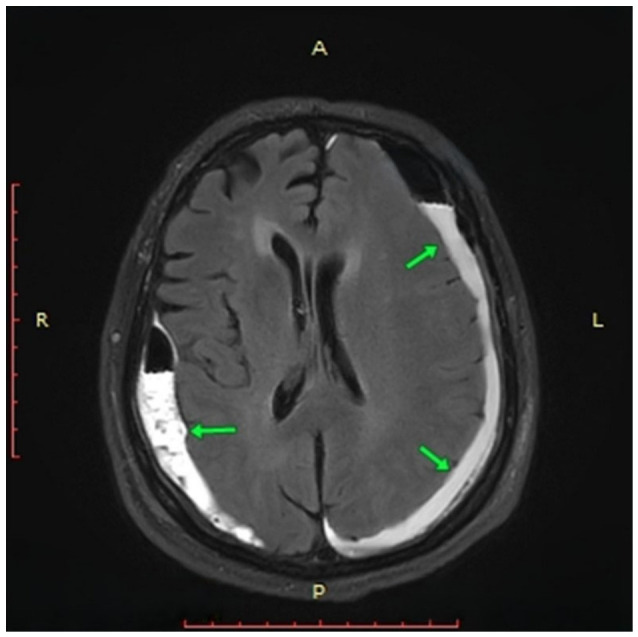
Axial section of the brain in T2 FLAIR sequence on 3T MRI (radiological image of hyperintense bilateral chronic subdural hematomas indicated by arrows). Own material. A—Anterior; L—Left; P—Posterior; R—Right.
